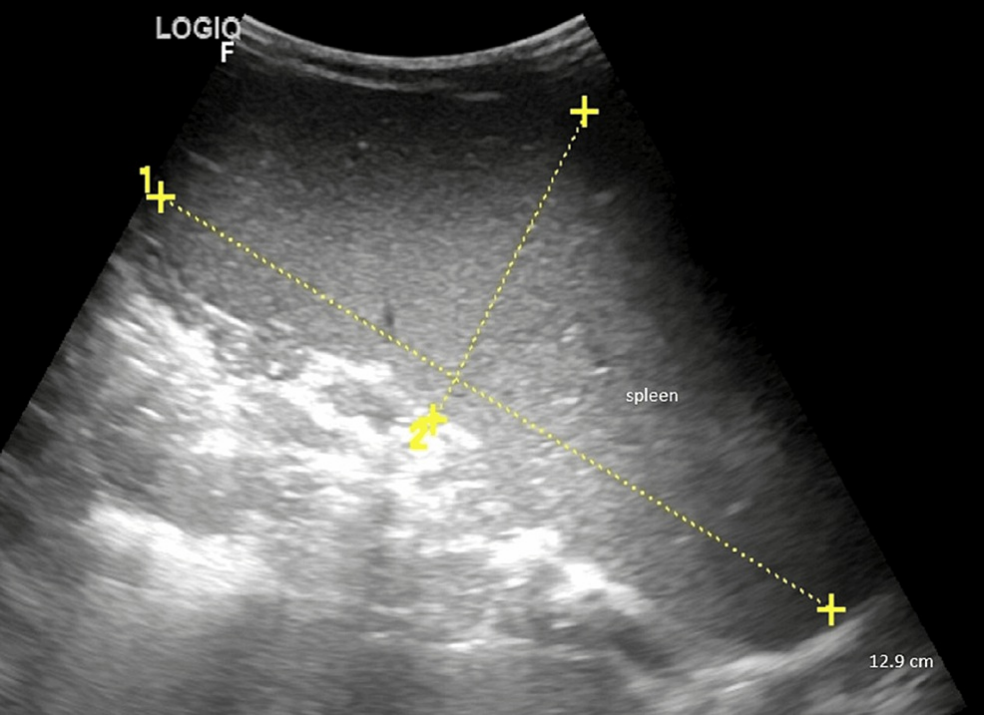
Ultrasonography of the abdomen showed hepatomegaly and splenomegaly of 12.9 cm (N<7)
